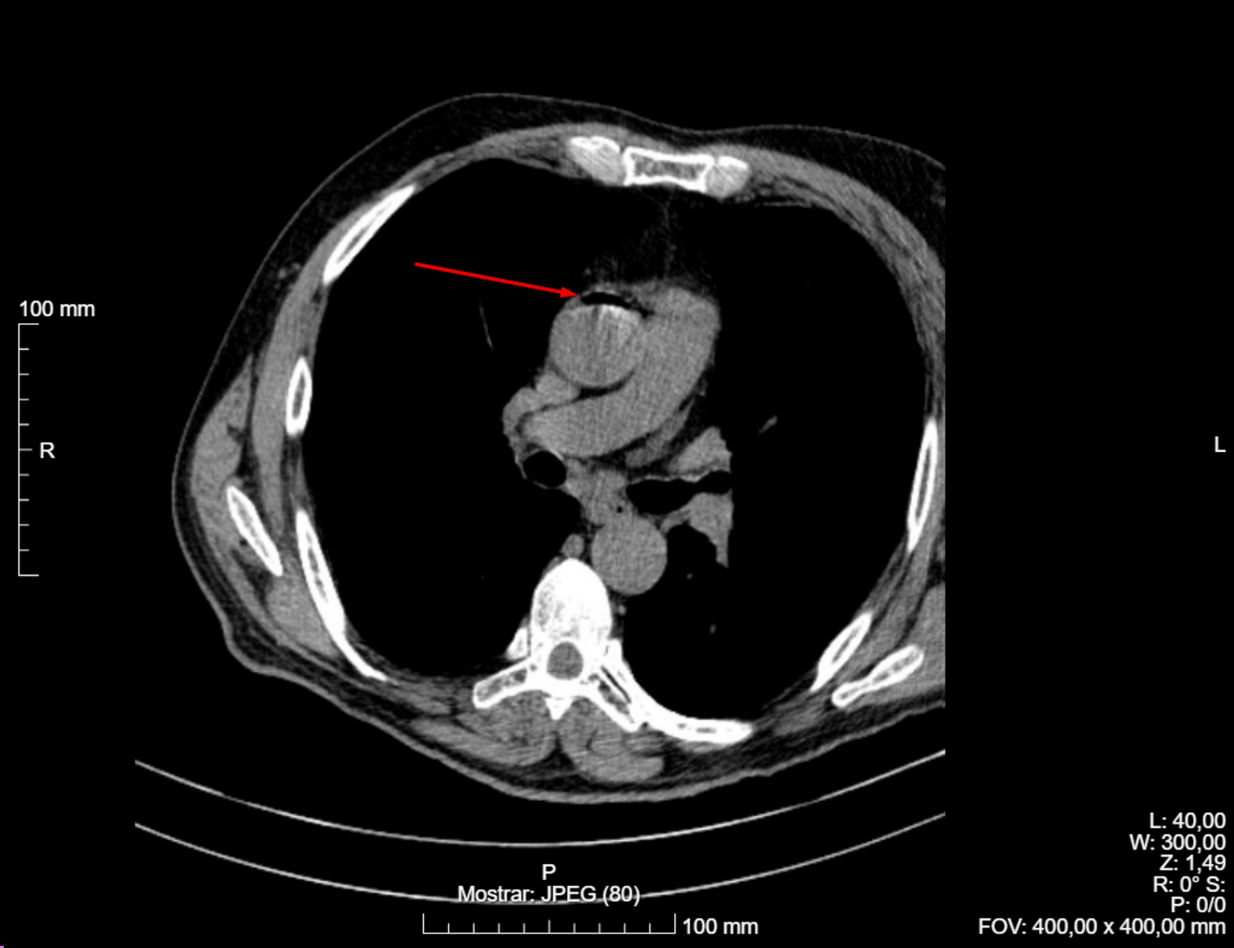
Control CT scan showing vascular (aorta) air embolism.
